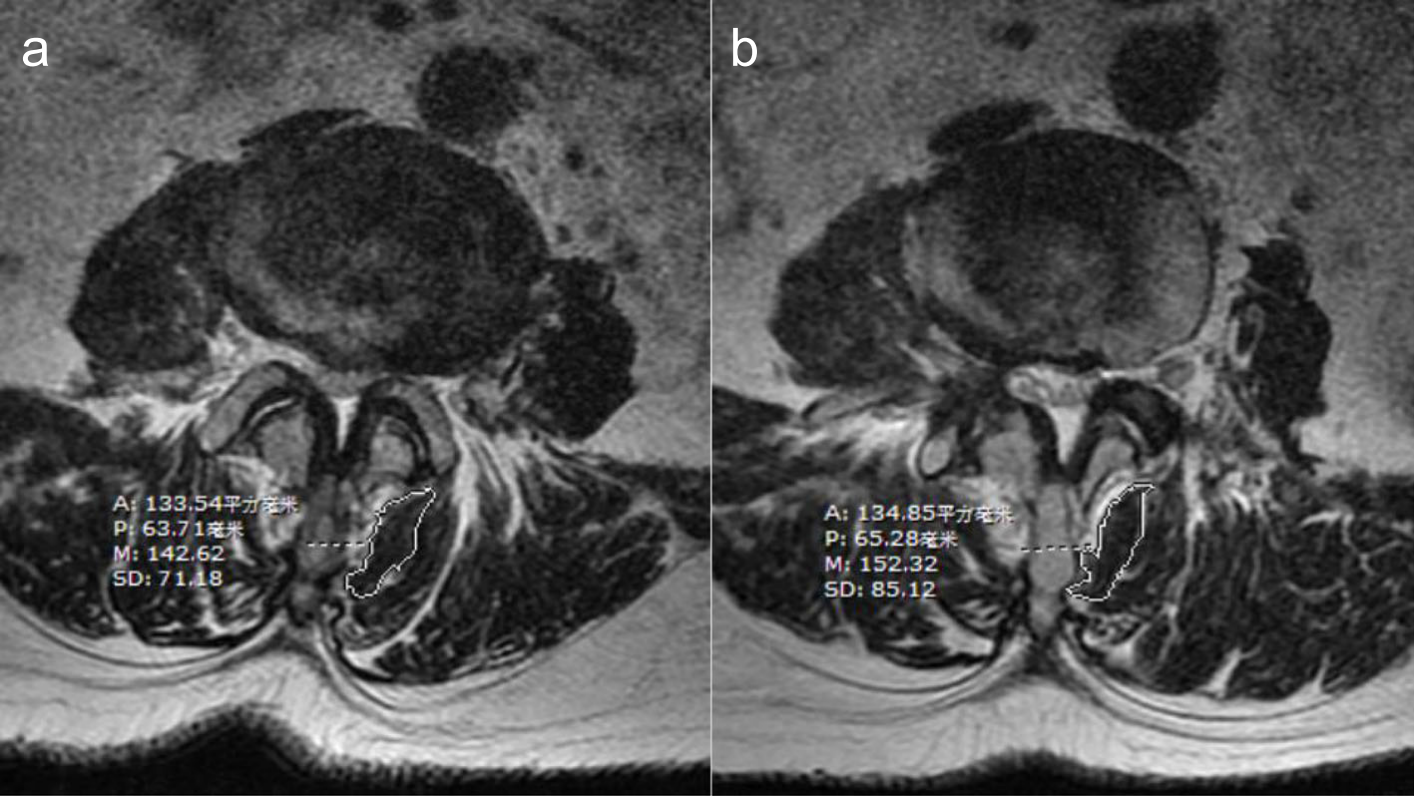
Calculation method of the CSA of the lumbar multifidus muscle on MRI. Note: The Philips MRI machine uses T2-weighted phase imaging of the L4-5 axial intervertebral space level of the lumbar spine surgery segment to select the cross-sectional boundary of the multifidus muscle by freehand tracing, and the system generates area values for area calculations. a (left) Patient, female, 58 years old, L4-5 intervertebral disc herniation, preoperative L4-5 disc level multifidus muscle CSA tracing method and area value (133.54 mm^2^). b (right) The method and area value (134.85mm^2^) of the CSA of the multifidus muscle in the L4-5 disc 6 months after surgery. A represents the CSA of the multifidus muscle (mm^2^); P indicates the perimeter of the scribed area (mm); M indicates the average gray value of the scribed area; SD indicates the standard deviation of the gray value of the scribed area
